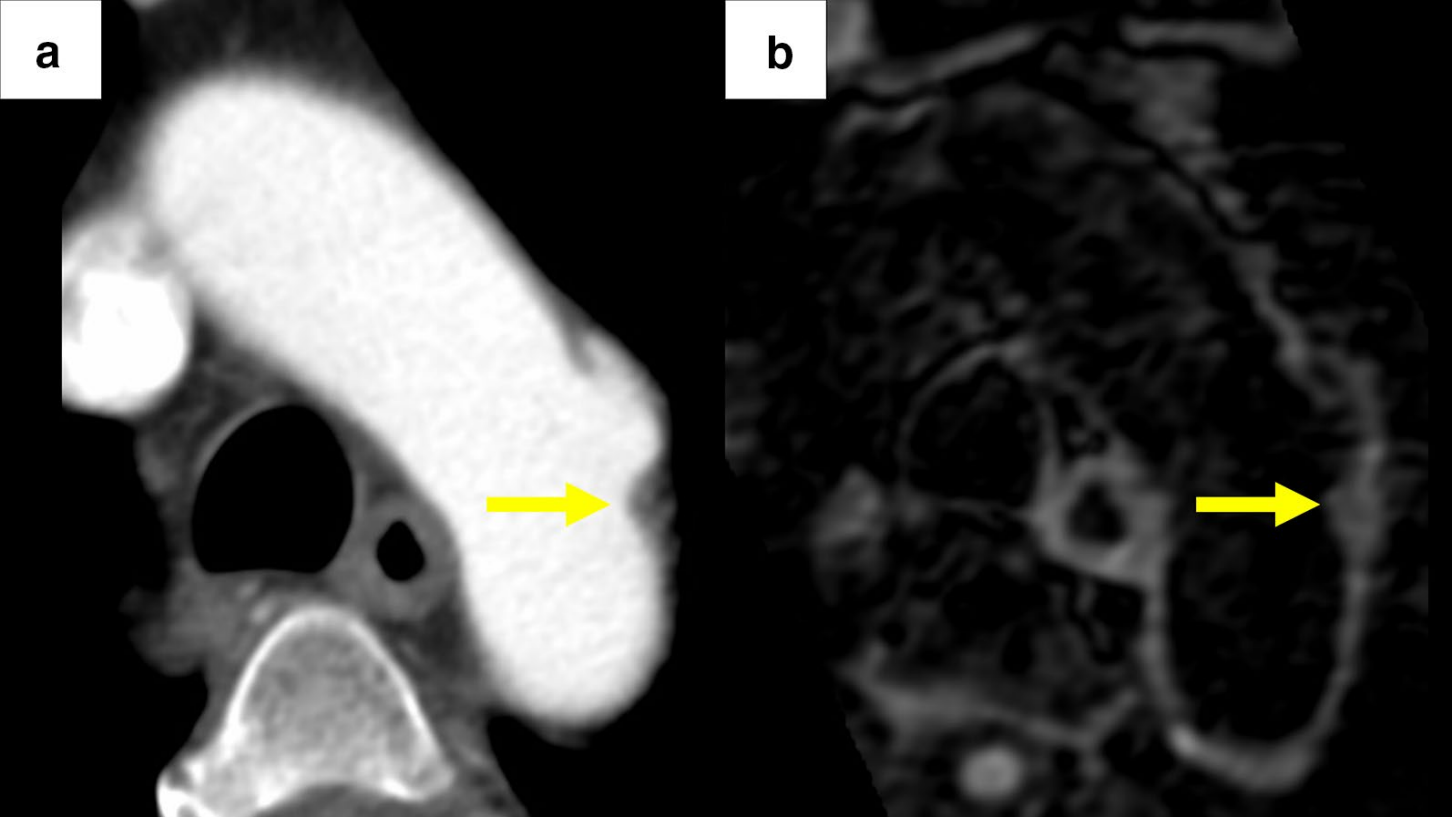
There is a small saccular aortic aneurysm on the distal arch with the atheromatic plaque. The saccular aneurysm was approximately 35 mm in diameter and the atheromatic plaque thickness was approximately 4 mm. a Computed tomography angiogram images and b magnetic resonance images
